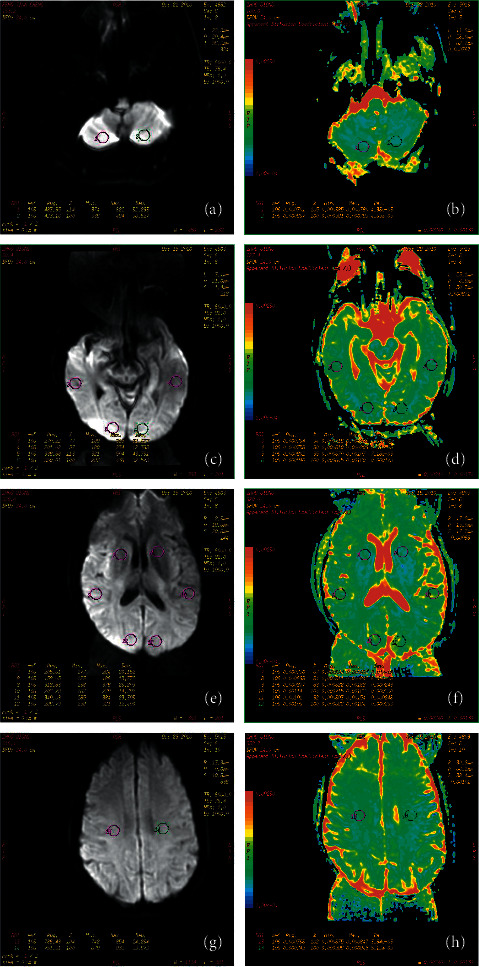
Diffusion-weighted imaging of each plane of the brain before surgery (a) Plane of cerebellum DWI (b) Plane of cerebellum ADC (c) Basal ganglia plane DWI (d) Basal ganglia plane ADC (e) Occipital plane DWI (f) Occipital plane ADC (g) Parietal plane DWI (h) Parietal plane ADC.
